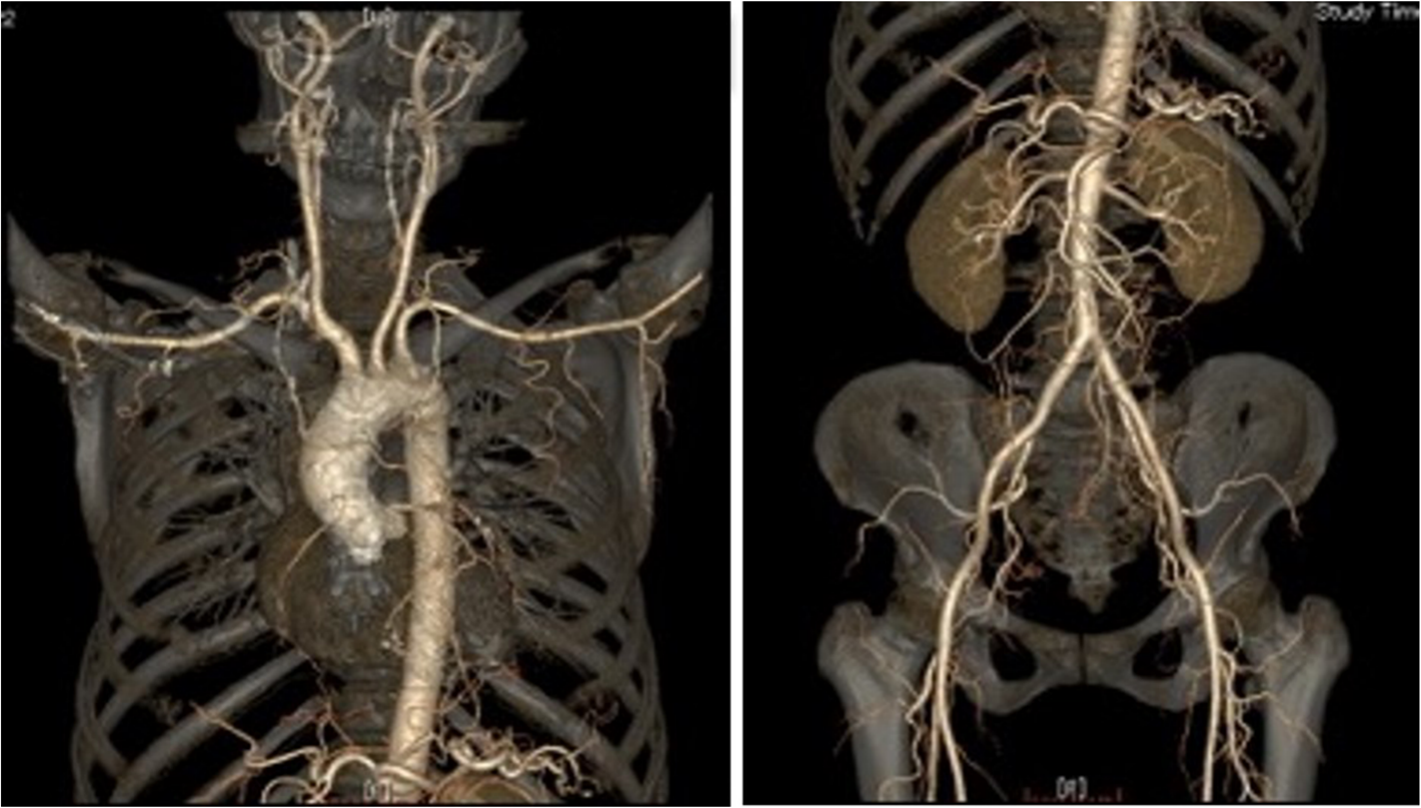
CT angiography. Systemic CT angiography revealed no stenotic or aneurysmal changes of arteries except for coronary arteries
